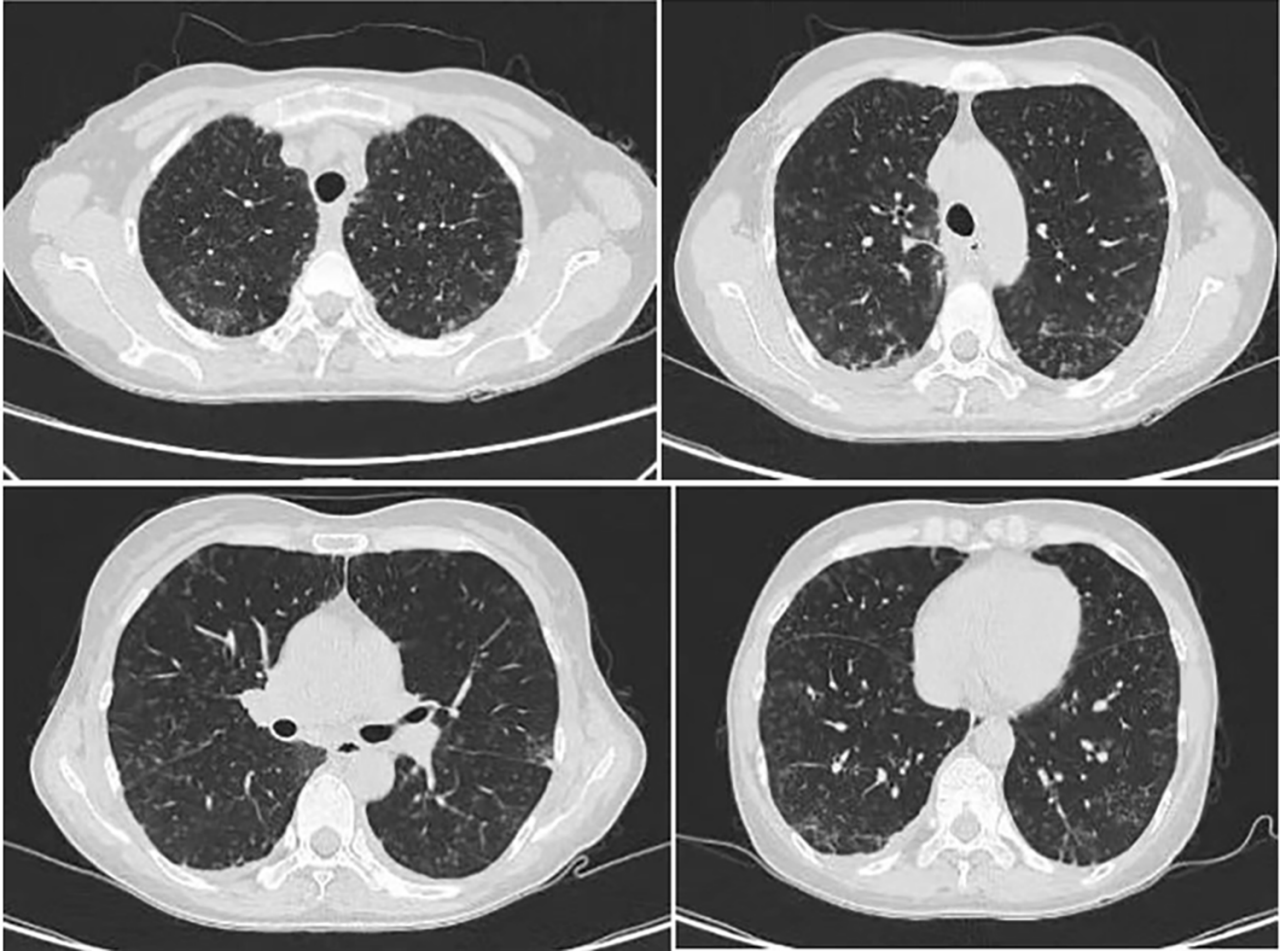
Chest computed tomography scans of immunocompetent patient in China who was co-infected with COVID-19 and non-HIV severe *Pneumocystis jirovecii* pneumonia, performed on June 25, 2023. Images show multiple tree-in-bud signs, ground-glass shadows, and substantially increased bilateral subpleural consolidation in the lungs.
